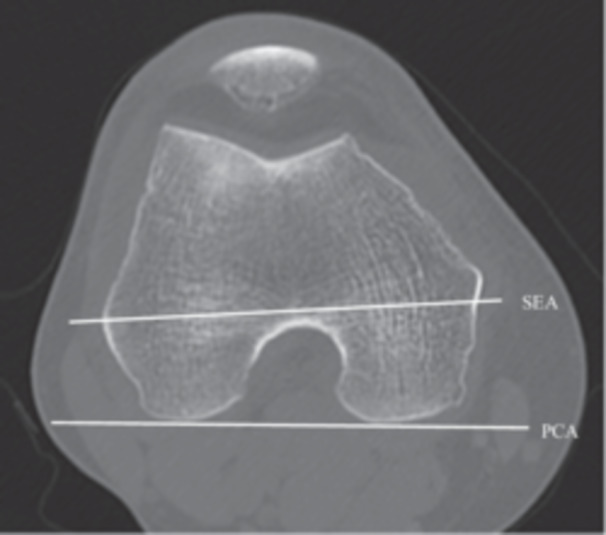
In the coronal slice of the CT image, the slice containing the lowermost point of the femoral posterior condyle was selected, and the line connecting the lowermost points of the medial and lateral posterior condyles was defined as the PCA. In addition, a slice was selected in the vicinity of the slice where the grooves of the lateral femoral epicondyle and medial femoral epicondyle could be seen, and the line connecting them was defined as the SEA. CT, computed tomography; PCA, posterior condylar axis; SEA, surgical epicondylar axis.
